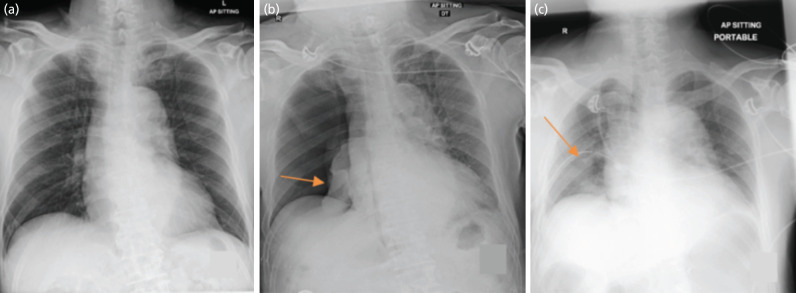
(a) Pre-operative chest radiograph – normal lung field expansion with no visible bullae noted. (b) Post-operative chest radiograph – right sided pneumothorax seen (indicated by arrow). (c) Chest radiograph post chest tube insertion (indicated by arrow) – with resolution of right sided pneumothorax seen.
